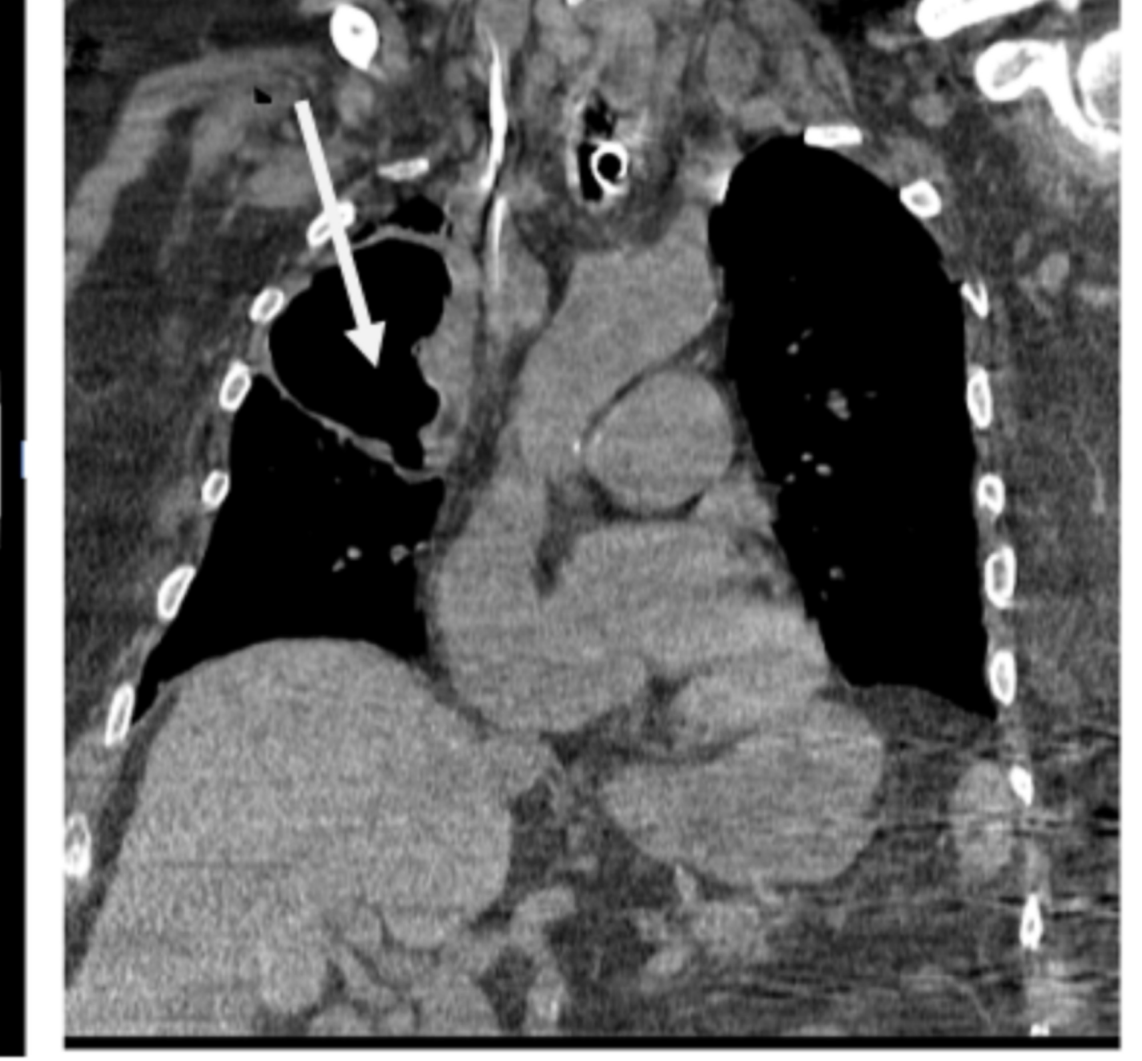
Chest CT scan: The pulmonary parenchyma demonstrates a large, thick-walled area of cavitation in the right upper lobe, measuring 7.3 x 5.4 x 7.5 cm. Multiple irregular nodules, quite small, less than 3 mm, peripheral to this area of cavitation in the right apex can also be seen.
